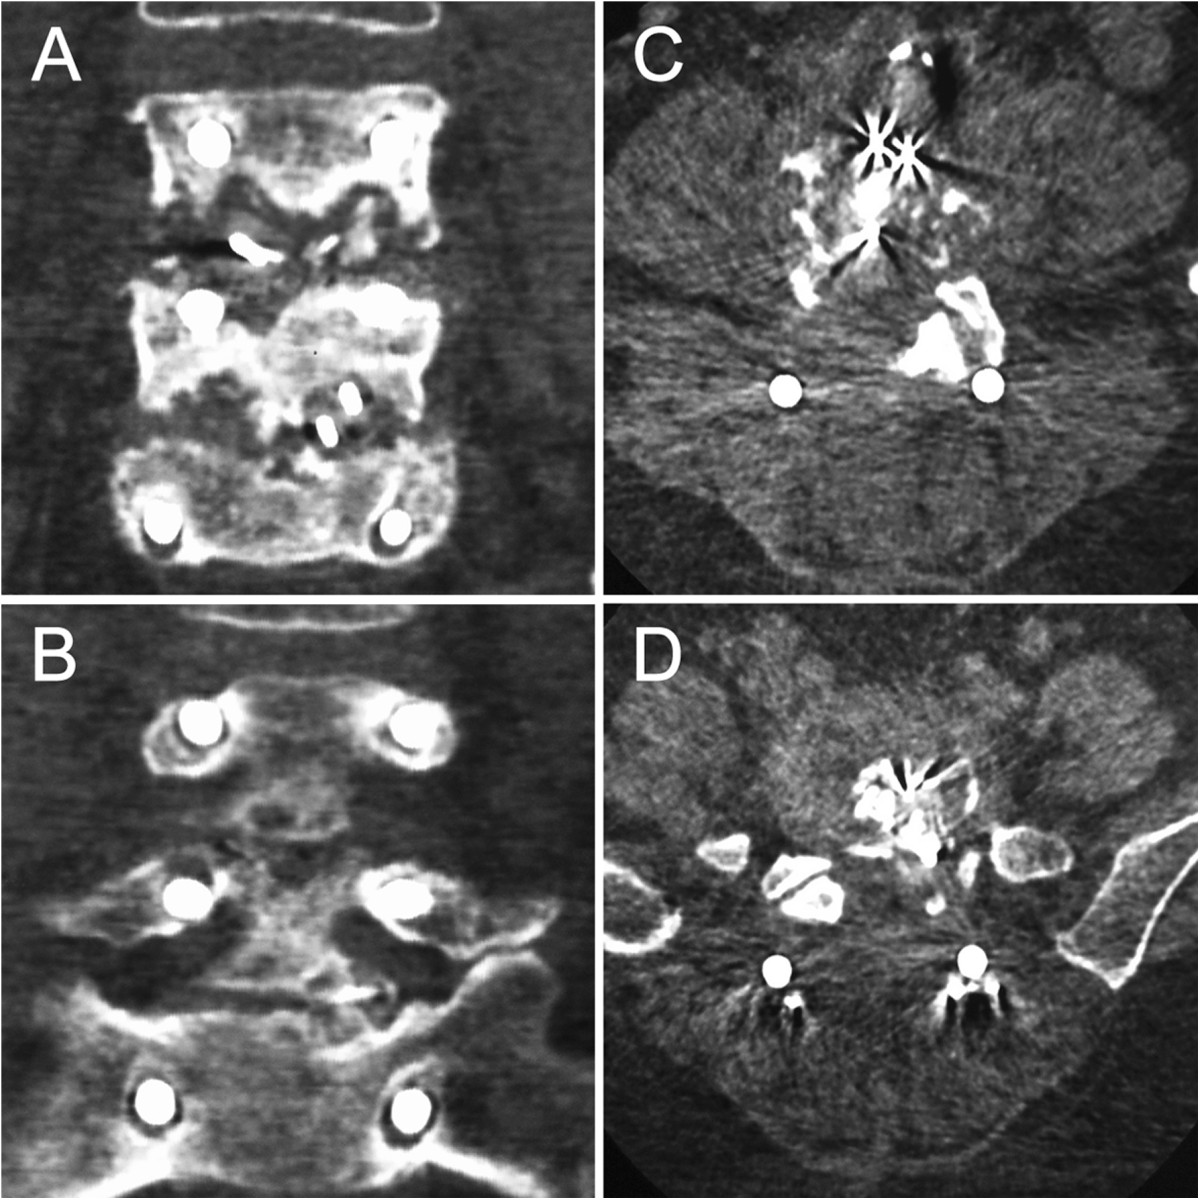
Musculoskeletal and fixator changes. Coronal, Computed tomography (CT) showing loosening of the pedicle screws and the osteolysis (A, B). Axial view indicating metallosis in the intervertebral space, the erector spinae with lower attenuation is also identified (C, D).
